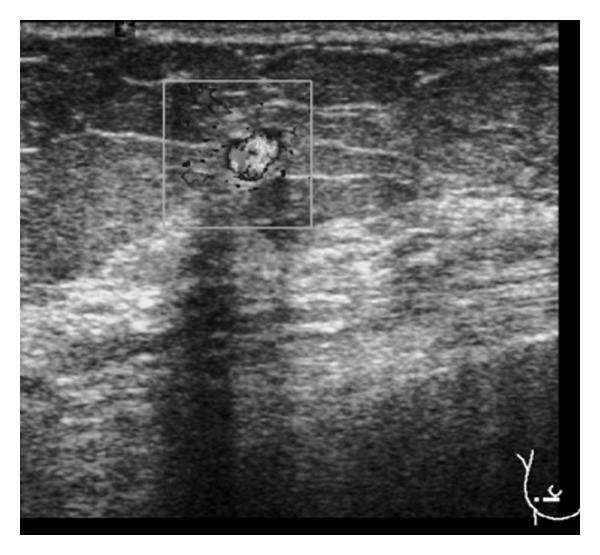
Hypoechoic, mass with lobular contours is observed hypervascular on color Doppler US at the lower outer quadrant at the right breast.
